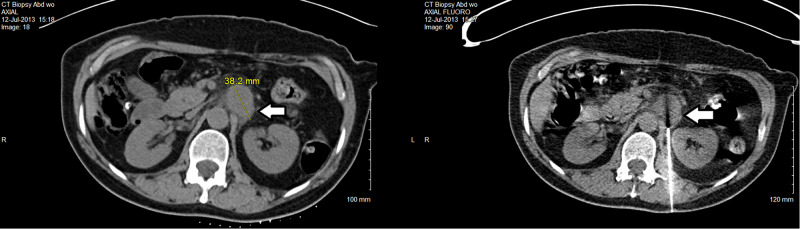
CT-guided core biopsy in July 2013 confirmed recurrent moderately differentiated adenocarcinoma (white arrow).
